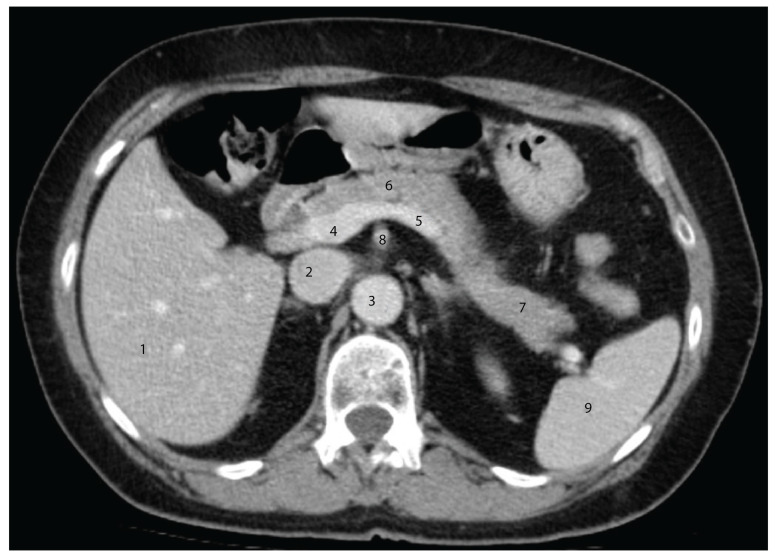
Axial CT section in the venous phase at the inferior border of the T12 vertebral body. 1—Right hepatic lobe; 2—inferior vena cava; 3—descending abdominal aorta; 4—portal vein; 5—splenic vein; 6—body of the pancreas; 7—tail of the pancreas; 8—superior mesenteric artery; 9—spleen.
